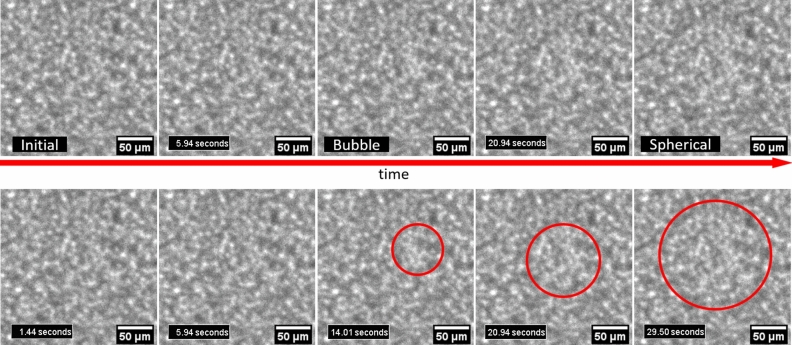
X-ray images from different times during the irradiation of a sludge-filled cuvette showing the same bubble forming. The images start from the earliest image on the left to increasingly later images on the right (1.4 s, 6 s, 14 s, 21 s, and 29.5 s with respect to the start of irradiation). The central panel shows the early signs of bubble formation around 15 s into the irradiation and the final panel shows the bubble has become spherical around 30 s into the irradiation. On the second row the images are reproduced with the approximate area considered to be occupied by the bubble marked in red to guide the eye. All images were of the same irradiation location and at the same scale. Figure made with images processed by FIJI^56^.
